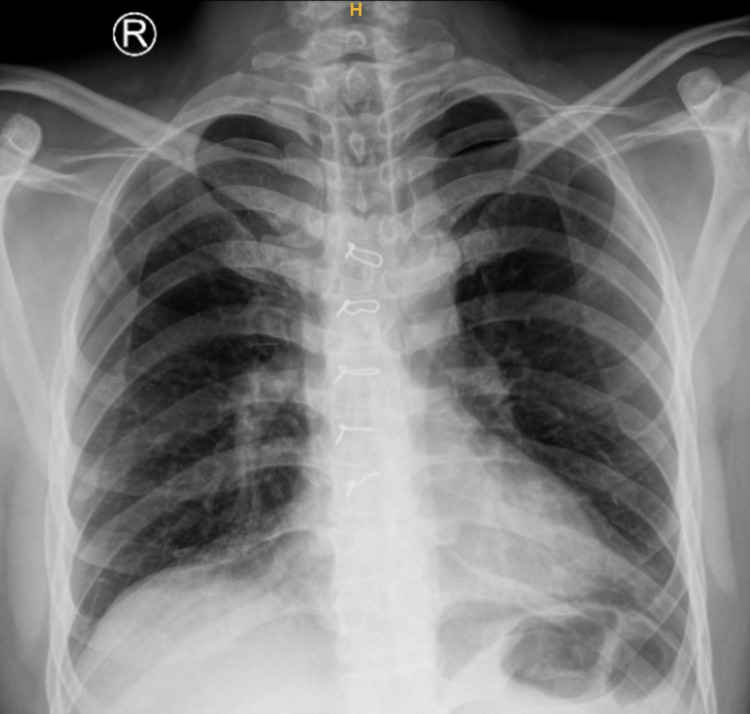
X-ray chest PA view. Prior surgical sternal wires are apparent. PA: posteroanterior.
